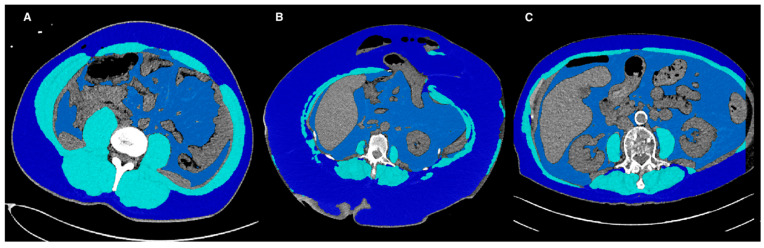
Example CT abdomen slices with TotalSegmentator-derived segmentation. Light blue = skeletal muscle; medium blue = visceral adipose tissue; dark blue = subcutaneous adipose tissue. Examples show (A) correct segmentation, (B) incorrect segmentation due to an abdominal wall hernia, and (C) incomplete segmentations due to incomplete coverage of the left lateral abdomen.
